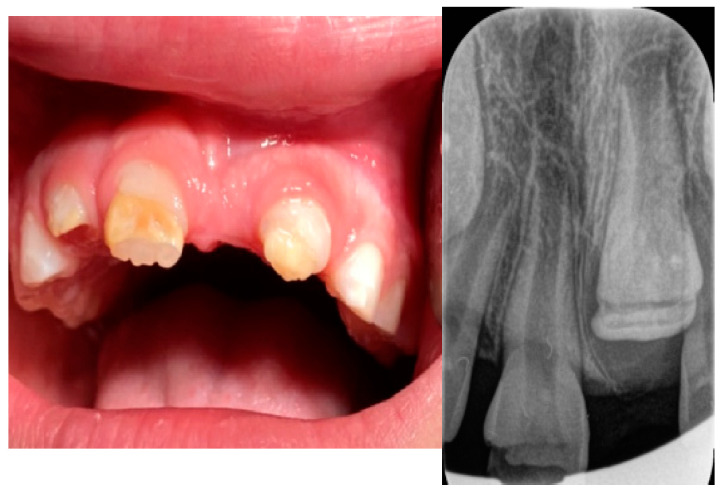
Intraoral view and radiography in March 2023.
